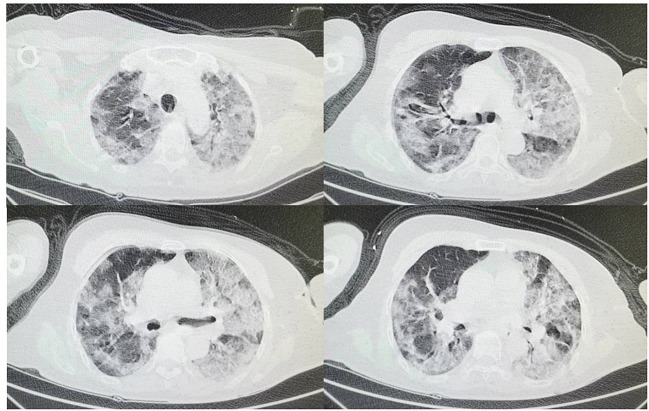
On February 5th at admission chest CT shows bilateral interstitial ground glass-like shadows and multiple consolidations.
